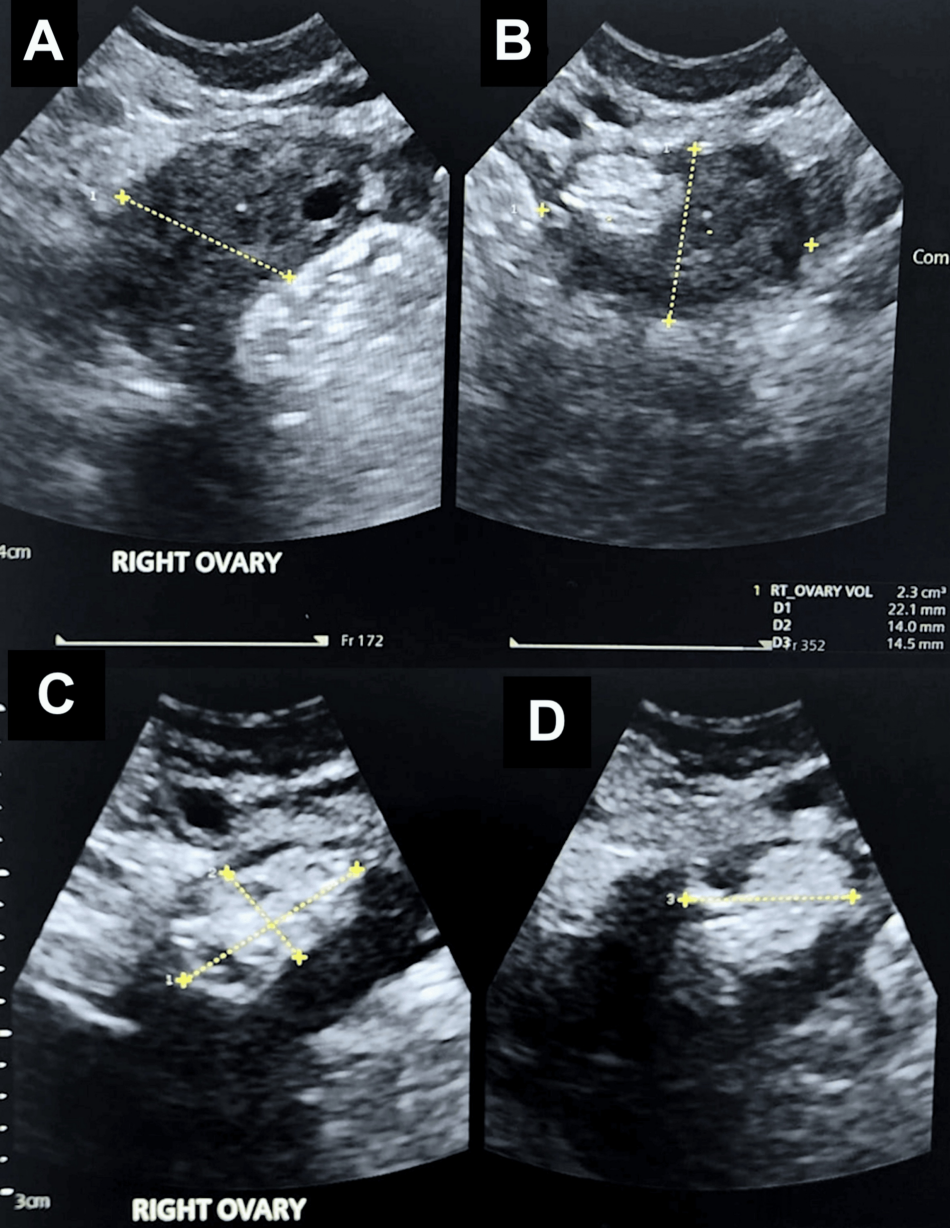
Transvaginal ultrasound of the right ovary demonstrating a suspected right ovarian dermoid cyst (A–B) Right ovary. (C–D) A hyperechoic, solid-appearing cyst containing multiple tiny cystic areas, measuring 12.9 x 6.9 x 10.5 mm, with no vascularity demonstrated on colour Doppler. The appearances are suggestive of a right ovarian dermoid cyst.
